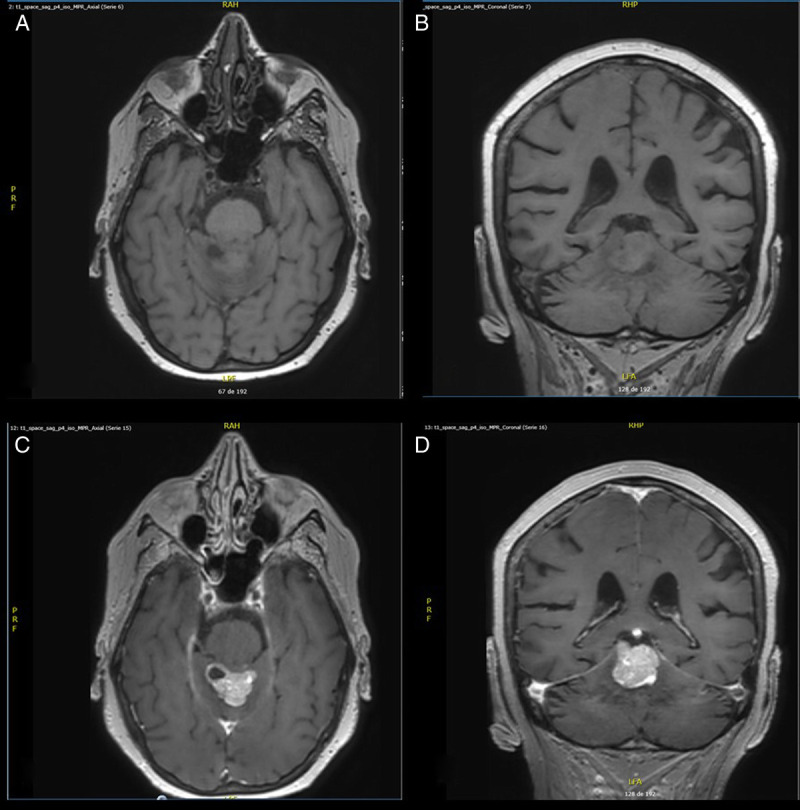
(A) Axial T1 weighted image without gadolinium. (B) Coronal T1 weighted image without gadolinium. (C) Axial T1 weighted image after gadolinium. (D) Coronal T1-weighted image after gadolinium showing an infratentorial lesion suspected for brain metastasis.
